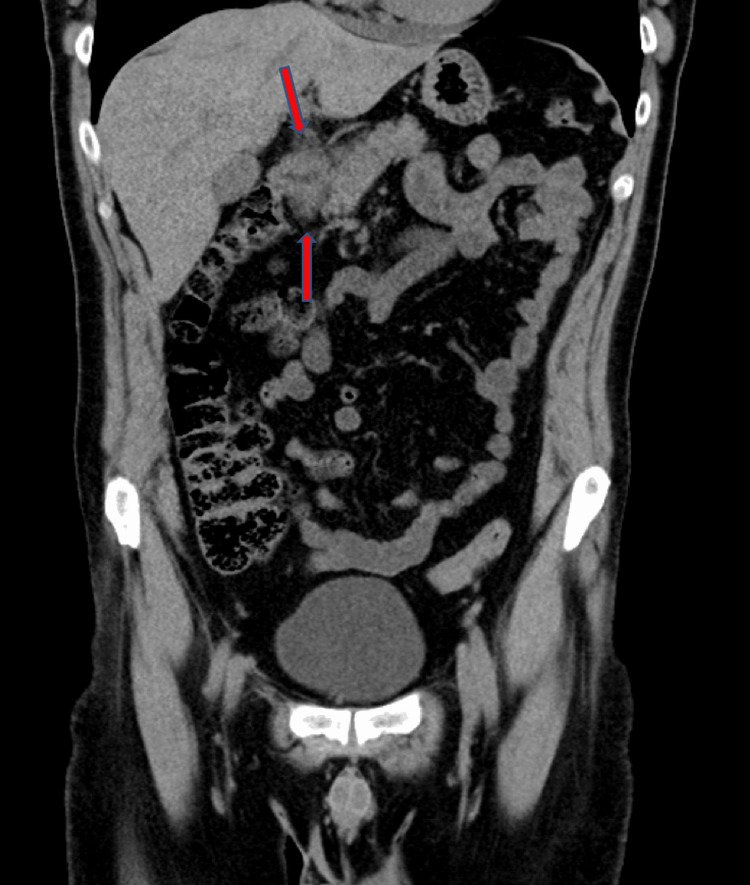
Magnetic resonance imaging of the abdomen. Coronal image demonstrating duodenal wall edema and thickening consistent with duodenitis (arrows).
